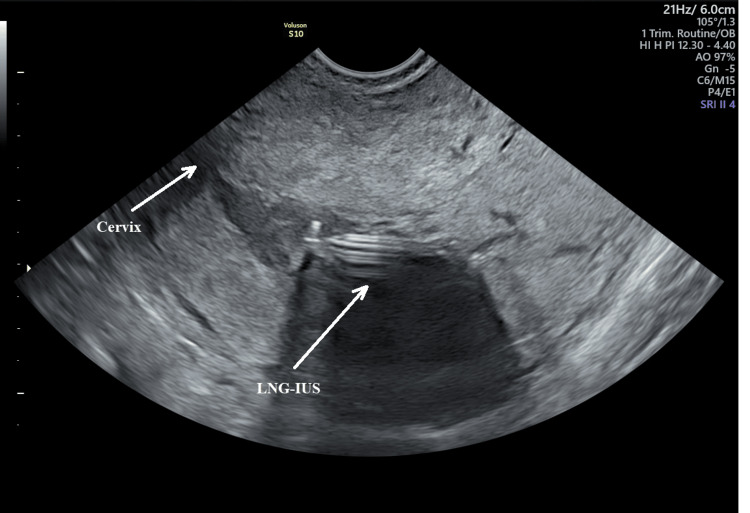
Ultrasound visualization of the correctly positioned levonorgestrel-releasing intrauterine system (LNG-IUS) adjacent to the gestational sac.
